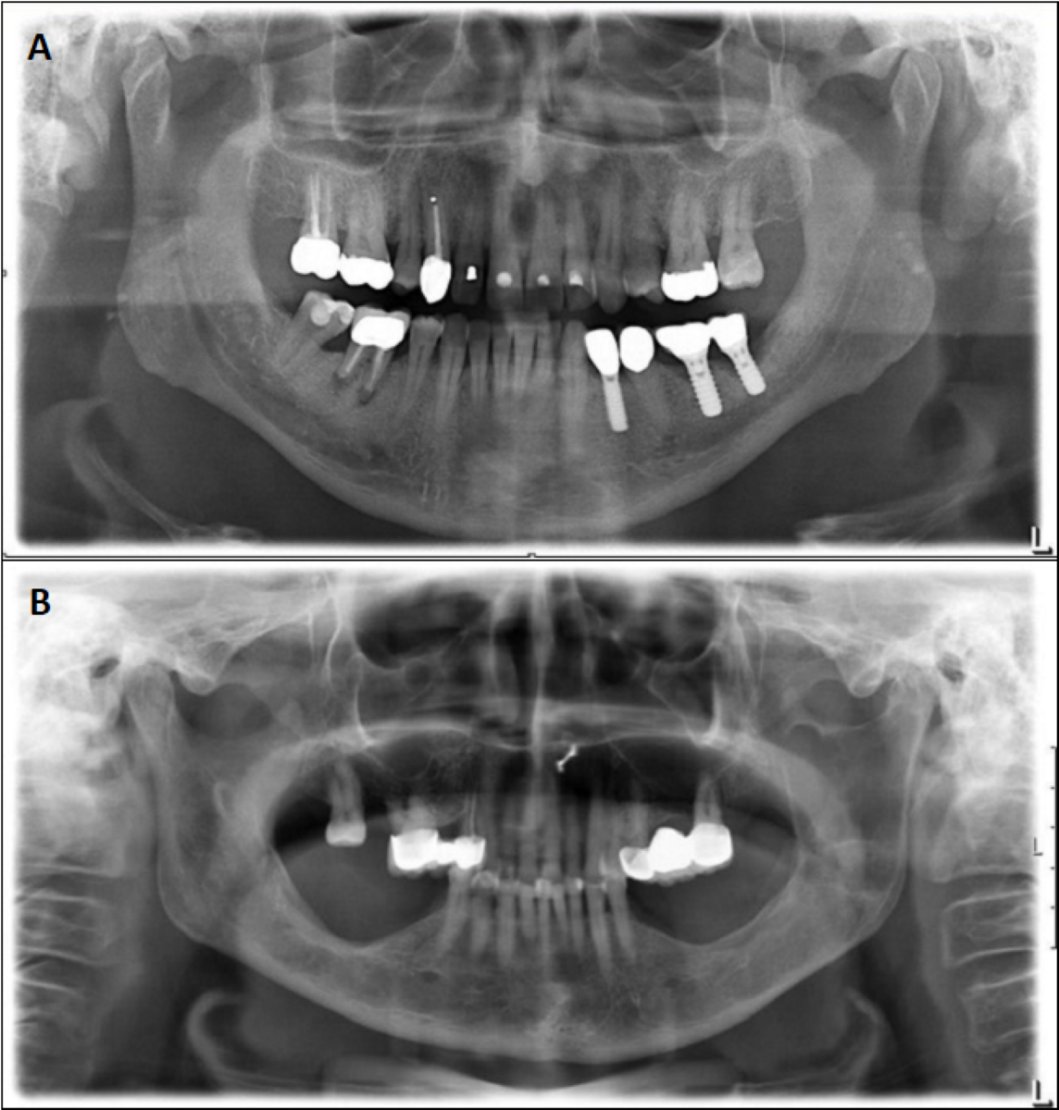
(A,B) Two randomly chosen panoramic radiographs featuring various pathologies to be diagnosed by dental students. Both x-ray images represented the basis of a student-written and an AI-generated radiology report. AI: artificial intelligence.
